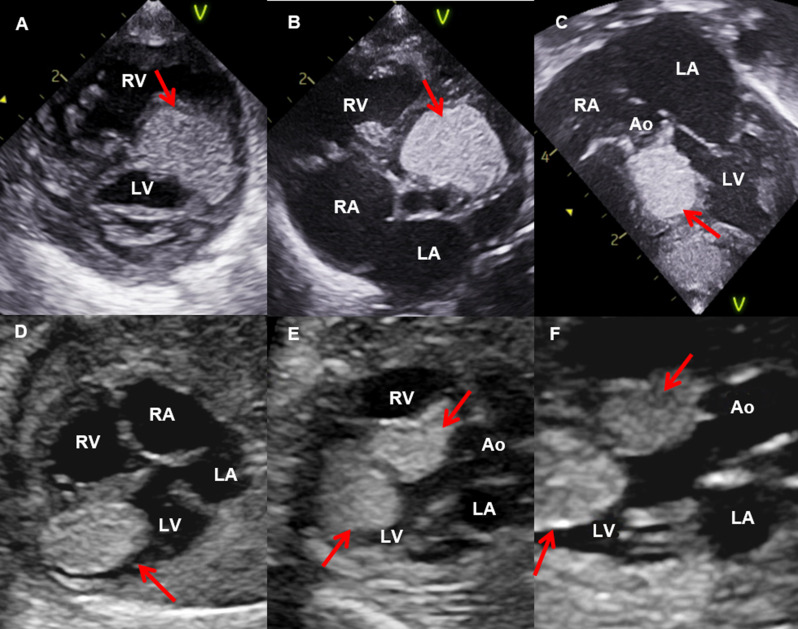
Cardiac rhabdomyomas observed by transthoracic and foetal echocardiography. In A, B, and C, transthoracic echocardiography images show an echogenic mass (arrows) adhered to the interventricular septum, invading and causing obstruction of the left ventricular outflow tract. These findings are demonstrated in the parasternal short-axis view of the ventricles (A), short-axis view of the aortic valve (B), and apical five-chamber view (C). In D, E, and F, foetal echocardiography images reveal echogenic masses (arrows) attached to the interventricular septum and the apical region of the left ventricle. These are shown in the four-chamber view (D) and five-chamber views (E and F). No obstruction of the left ventricular inflow or outflow tracts was observed, with preserved aortic valve opening (F). RA: right atrium; LA: left atrium; RV: right ventricle; LV: left ventricle; Ao: aorta. Exams performed at the Paediatric Echocardiography Department of the Heart Institute
